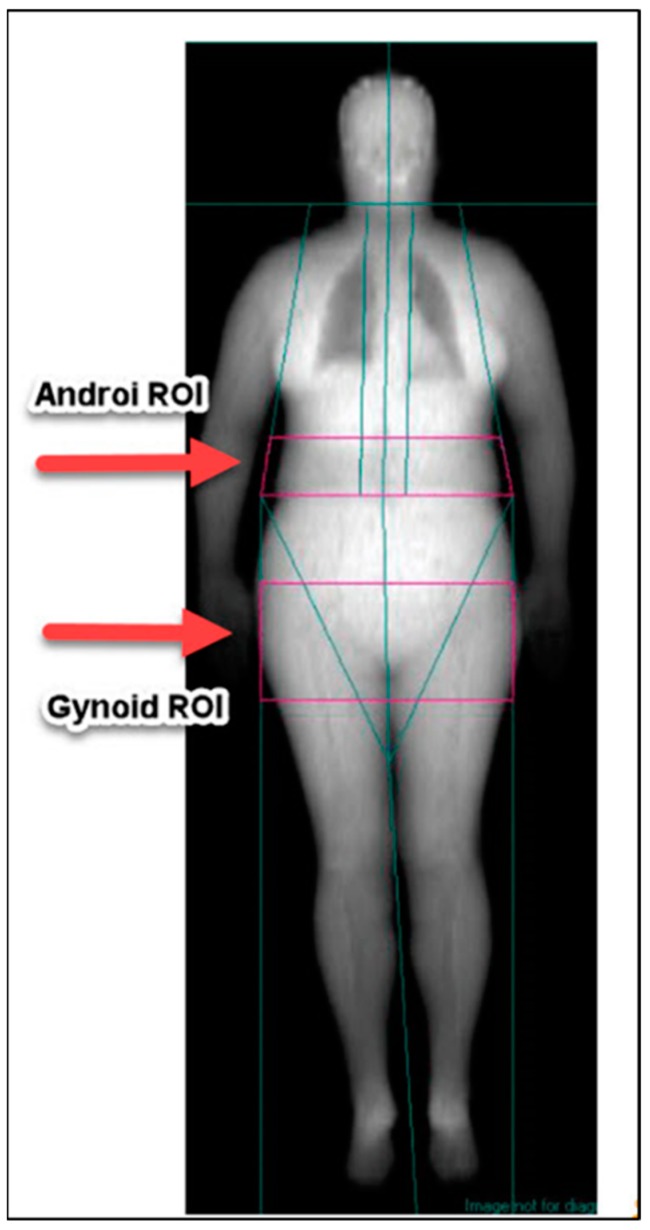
Example scan image with android (top arrow) and gynoid regions (bottom arrow) identified from the GE LUNAR iDXA Narrow-Angle Dual Energy X-ray Densitometer with SmartFAN™ (GE Medical, Software Lunar DPX enCORE 2012 version 14.0, Madison, WI, USA).
